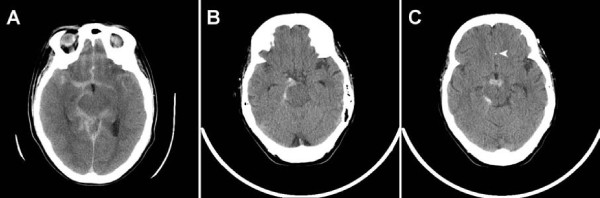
The patient's non-contrasted head computed tomography scan (A) at the time of her initial aneurysmal subarachnoid hemorrhage admission and (B and C) subsequent non-aneurysmal subarachnoid hemorrhage revealing perimesencephalic hemorrhage. The superior aspect of the coil mass from her previously treated anterior communicating artery aneurysm, remote from the new non-aneurysmal subarachnoid hemorrhage, is noted (C, arrowhead).
